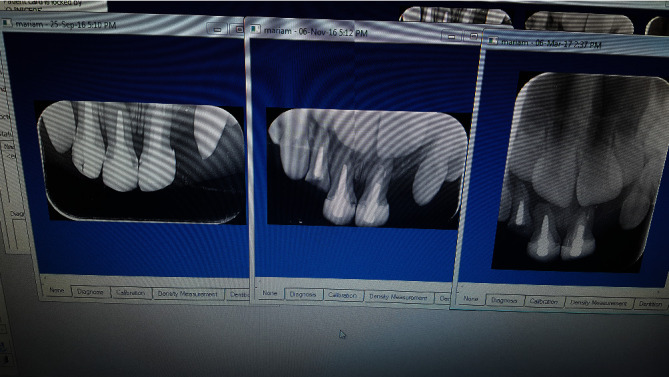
Radiographic follow-up of treated teeth.
